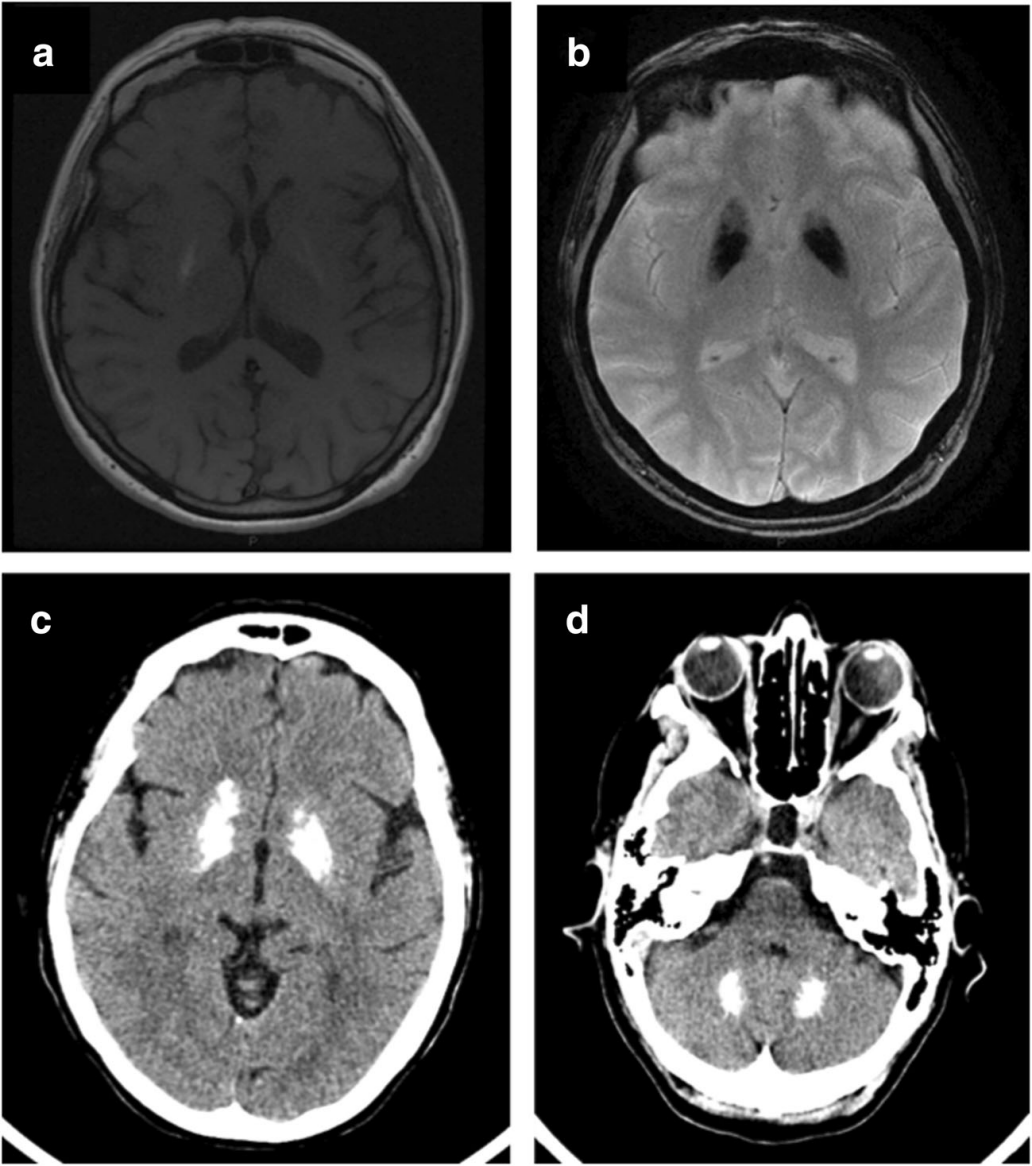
Brain MRI showing T1-w hyperintensity in basal ganglia (a) and severe T2*-GRE hypointensities in the same regions (b). Brain CT scan showing diffuse symmetric calcifications involving basal ganglia (c), and dentate nucleus (d). GRE gradient recalled echo, FLAIR fluid-attenuated inversion recovery
